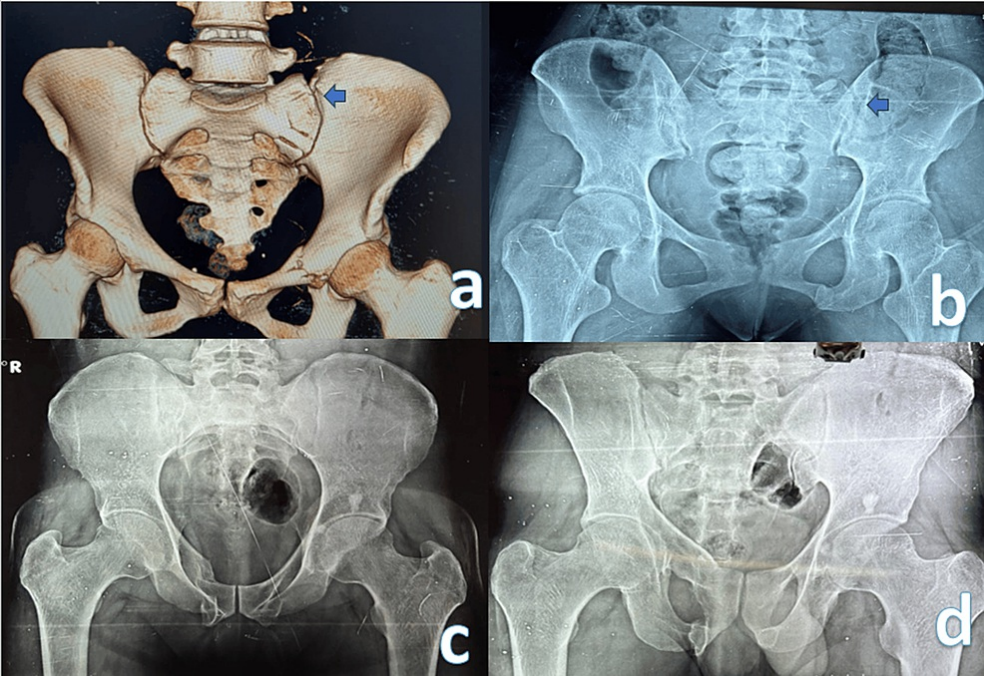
(A, B) Sacrum fracture Dennis type 1 managed conservatively. (C, D) One-year follow-up X-ray.
